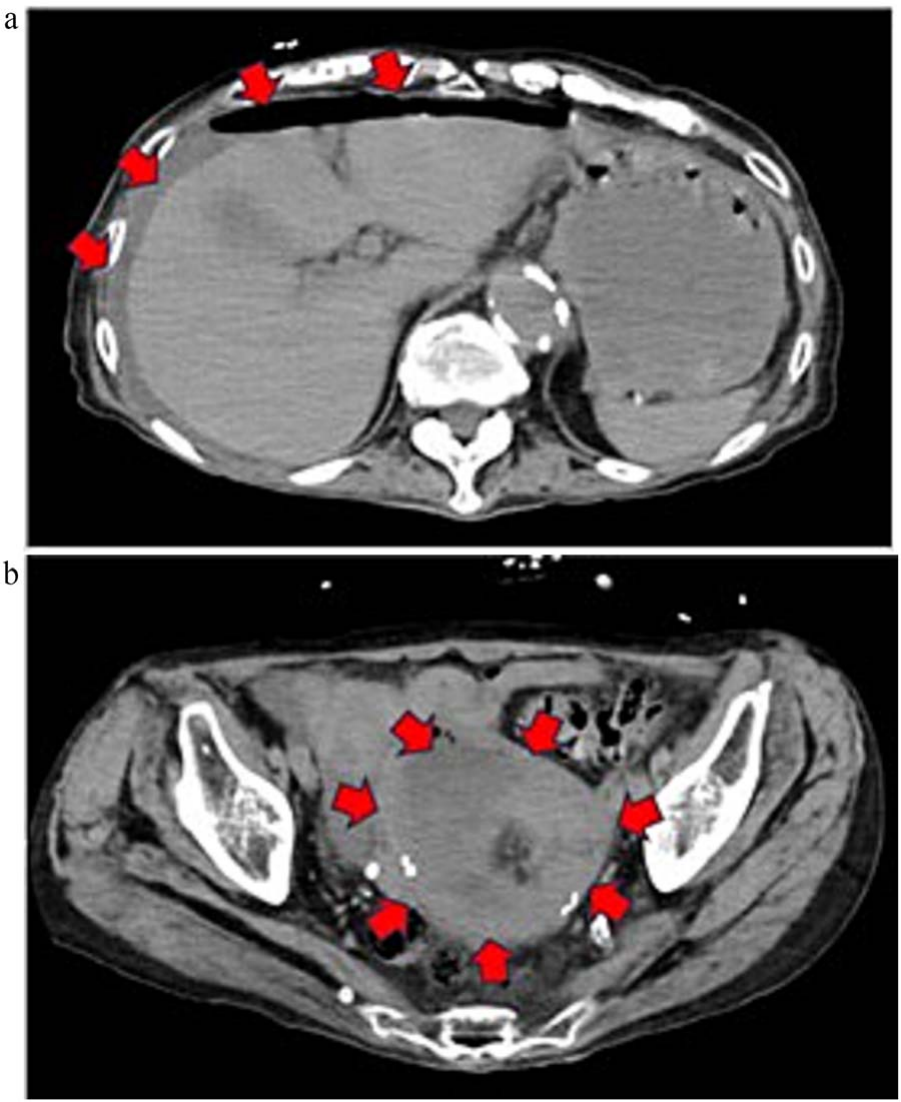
Preoperative contrast-enhanced CT image (equilibrium phase). (a) Horizontal section. Extensive ascites extending to the liver surface and free gas in the abdominal cavity are noted. (b) Horizontal section. A low-attenuation area suggestive of a retained uterine abscess was identified within the uterus.
